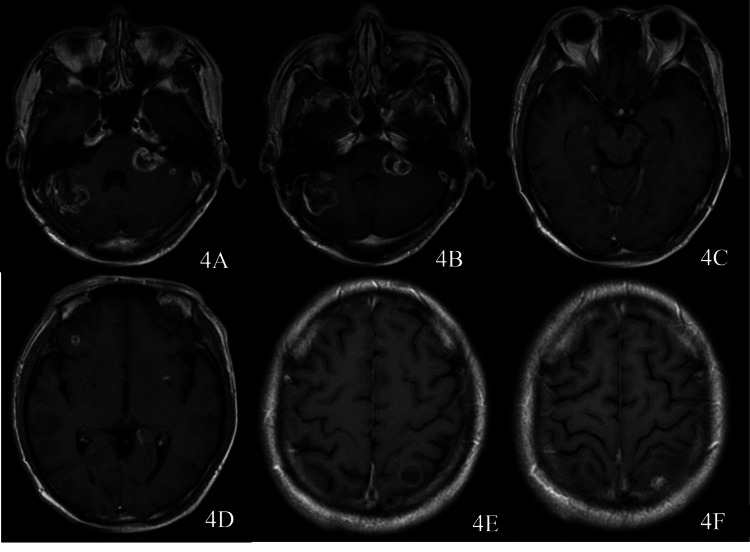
Findings at two years after the operation A magnetic resonance imaging (MRI) scan showing the tumors in the right cerebellar hemisphere (4A, 4B), the left brachium pontis, and the left cerebellopontine angle area to be substantially enlarged, and the ring to be enhanced (4A, 4B). The lesion of the left auditory nerve trunk is remarkably enlarged, the lesions in the right hippocampal area are slightly changed (4C), the enhanced signals in the left hippocampal area have disappeared, and new lesions can be seen in the right frontal lobe, left basal ganglia (4D), and left occipital lobe (4E, 4F).
